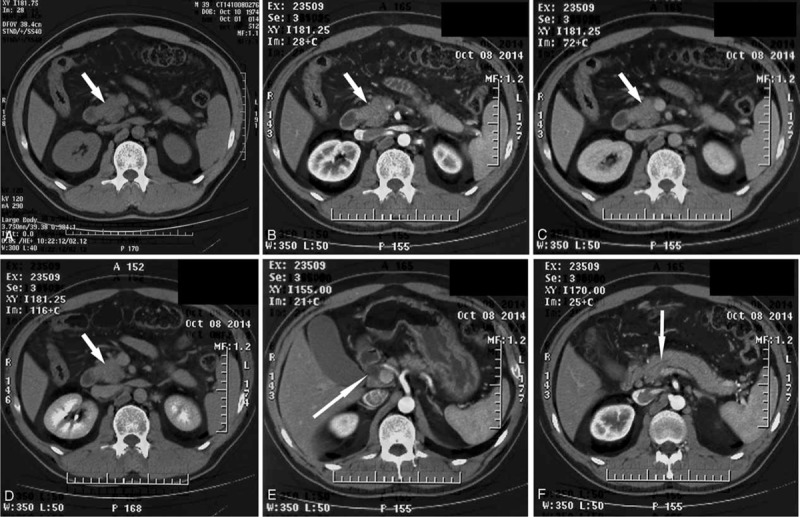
Abdominal CT demonstrates a low-density mass measuring 3.5 × 3.2 cm in the uncinate process of the pancreas, with dilated common bile and pancreatic ducts. (A) to (D) represent the plain phase, arterial phase, portal phase, and venous phase, respectively. (E) shows the dilated bile duct, and (F) reveals the dilated pancreatic duct.
